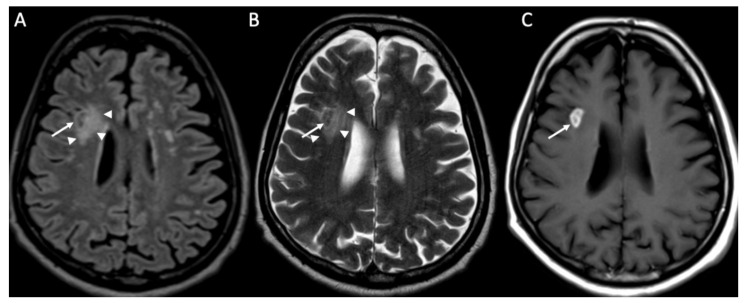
Axial FLAIR (A), T2 (B), and enhanced T1 (C) shows a tuberculoma in the right frontal region, which is hypointense in FLAIR and T2, and has intense contrast enhancement (arrows in A–C). Note also the surrounding vasogenic edema (arrowheads in A,B).
